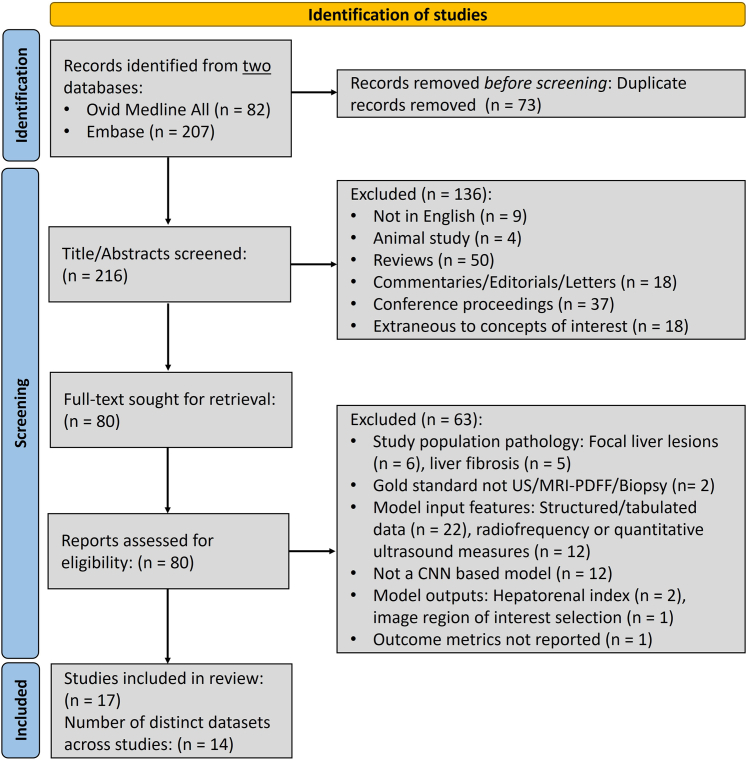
Study selection. Numbers are accurate as of Dec 12, 2023. US: Ultrasound, MRI-PDFF: Magnetic Resonance Imaging Proton Density Fat Fraction.
